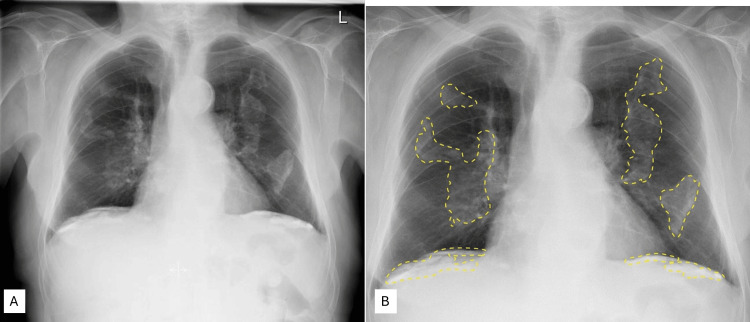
Chest radiographs of a 65-year-old male Image A shows calcified plaques on the anterior chest wall and the diaphragmatic surface. Image B shows the same chest X-ray, where the plaques are highlighted with yellow dotted lines User-generated image content in accordance with the CC License; https://radiopaedia.org/cases/12388 (rID number 12388)
